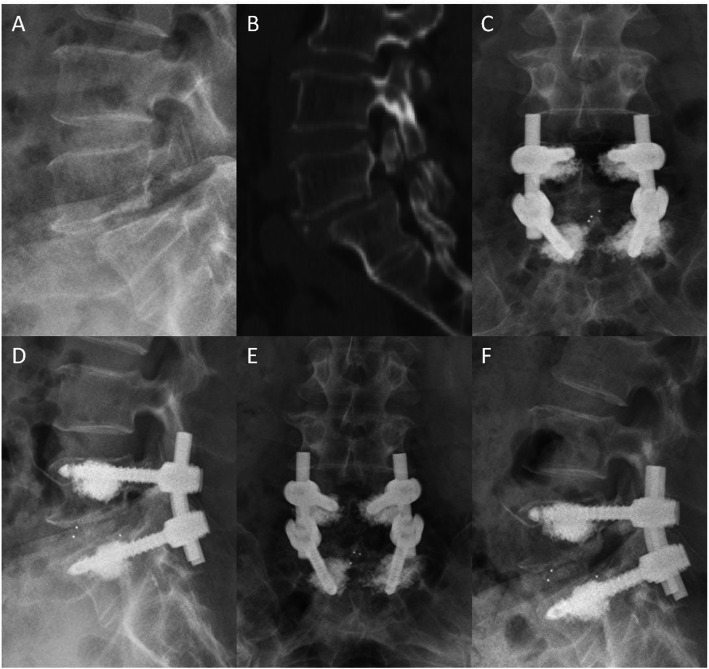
A 72-year-old female diagnosed with lumbar spondylolysis at the L5 vertebral body with osteoporosis (*T* = −2.8). a–b Preoperative lateral X-ray and CT scan showing grade II ture spondylolisthesis. c–d Bilateral PMMA-augmented CPSs are used for spinal fixation. The L5 vertebral body is well corrected, but PMMA leaked into the vertebral vein without any PMMA-related symptoms. e–f Lateral X-ray and CT scan at the last follow-up showing that no screw loosening occurs, and bony fusion is achieved. Lower back pain is ameliorated for this patient
